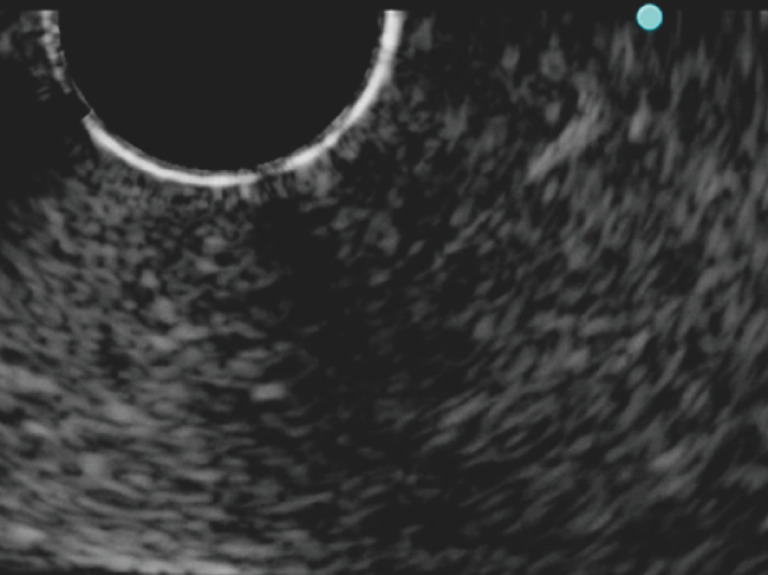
Ultrasound image showing that visibility of the puncture needle was poor when the high frequency transducer was rotated 10° counterclockwise.
